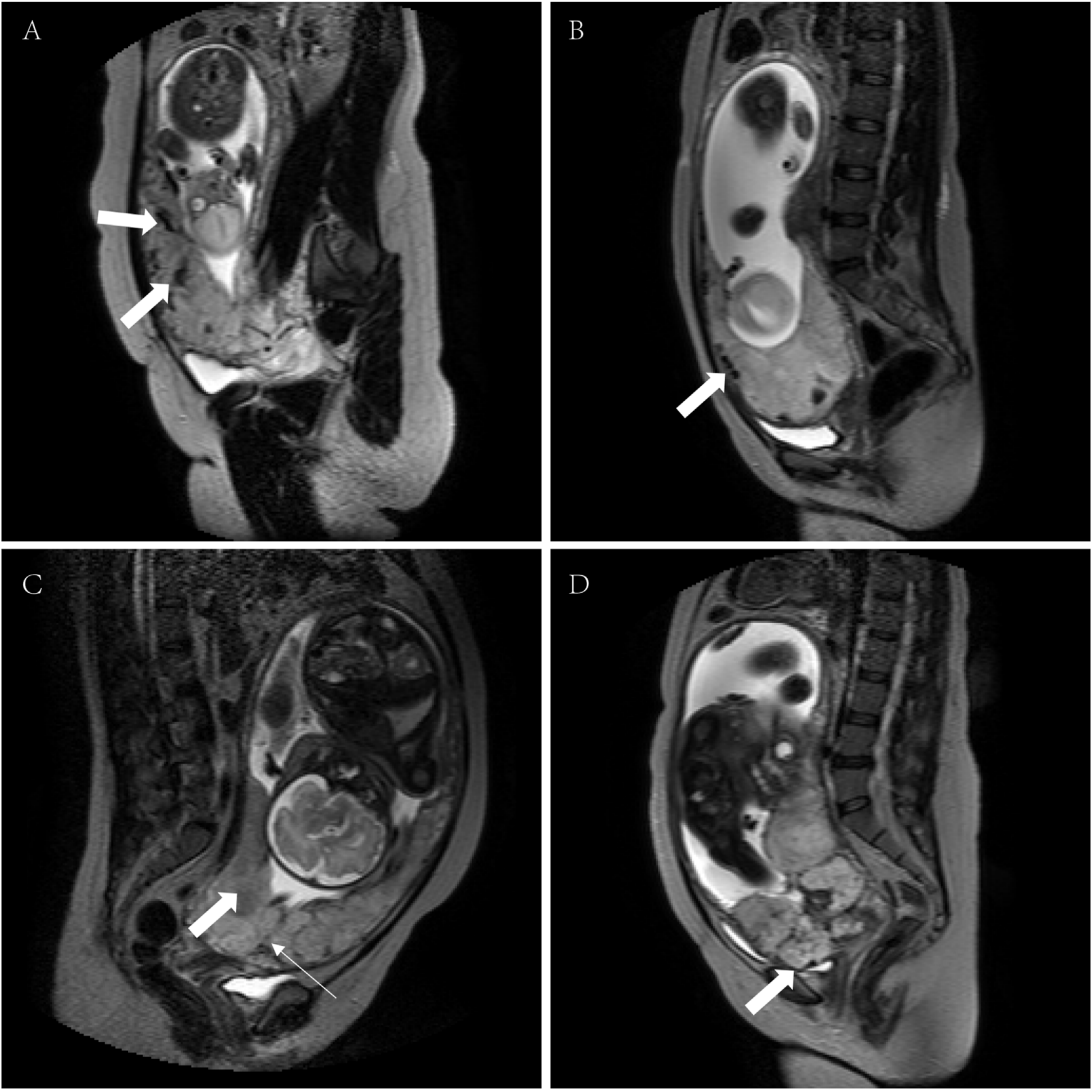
MRI signs associated with PPP (T2WI and sSSh-TSE sequences). (A) Intraplacental abnormal vascularity (arrows), (B) abnormal vascularization of the placental bed (arrow); (C) heterogeneous placenta (broad arrow) with a dark band on T2WI (narrow arrow); and (D) serosal surface of the bladder disruption (arrow). MRI, magnetic resonance imaging; PPP, pernicious placenta previa; T2WI, T2-weighted imaging; sSSh-TSE, Sense single-shot turbo spin echo.
